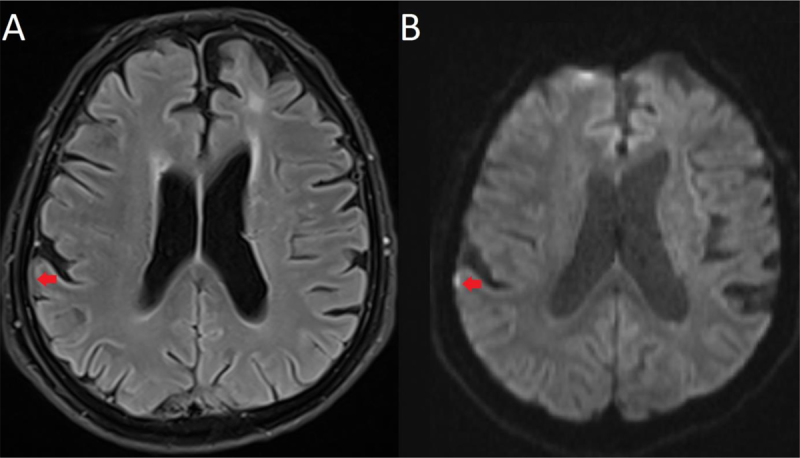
There was no ependymal enhancement nor meningeal thickening on gadolinium enhanced T1-weighted images.
